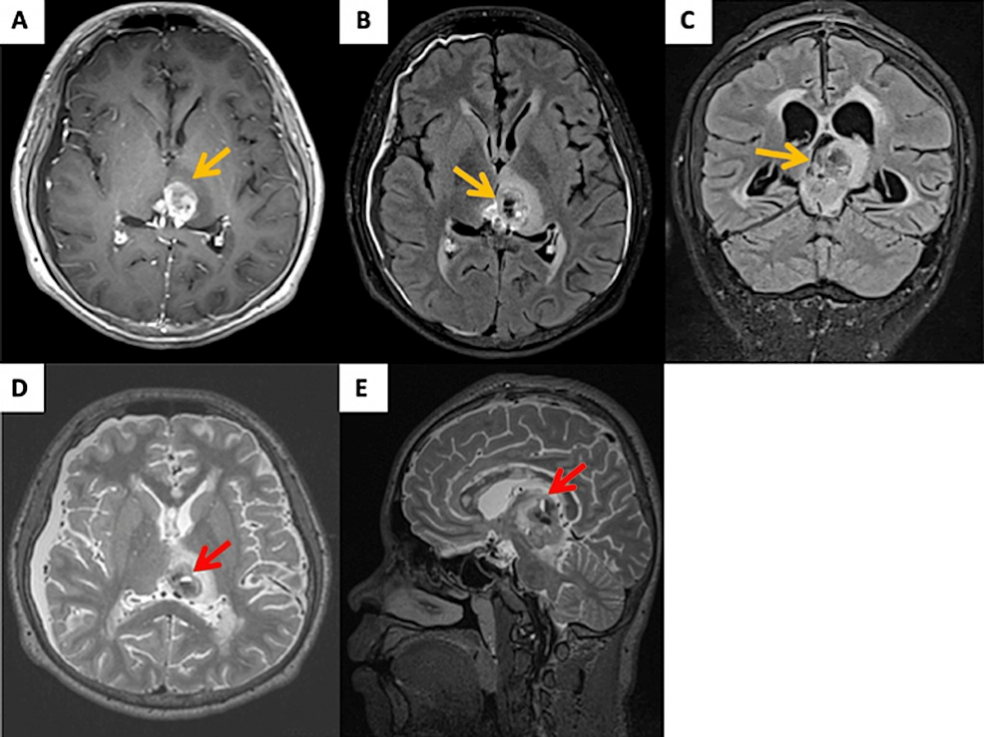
MRI images show a large pineal mass in (A) T1-contrasted axial cut (orange arrow), (B) T2-FLAIR axial cut (orange arrow), (C) T2-FLAIR coronal cut (orange arrow), (D) T2-contrasted axial cut with fluid level (red arrow) in tumor, and (E) T2 sagittal cut with fluid level (red arrow) in tumor. FLAIR, fluid-attenuated inversion recovery; MRI, magnetic resonance imaging
